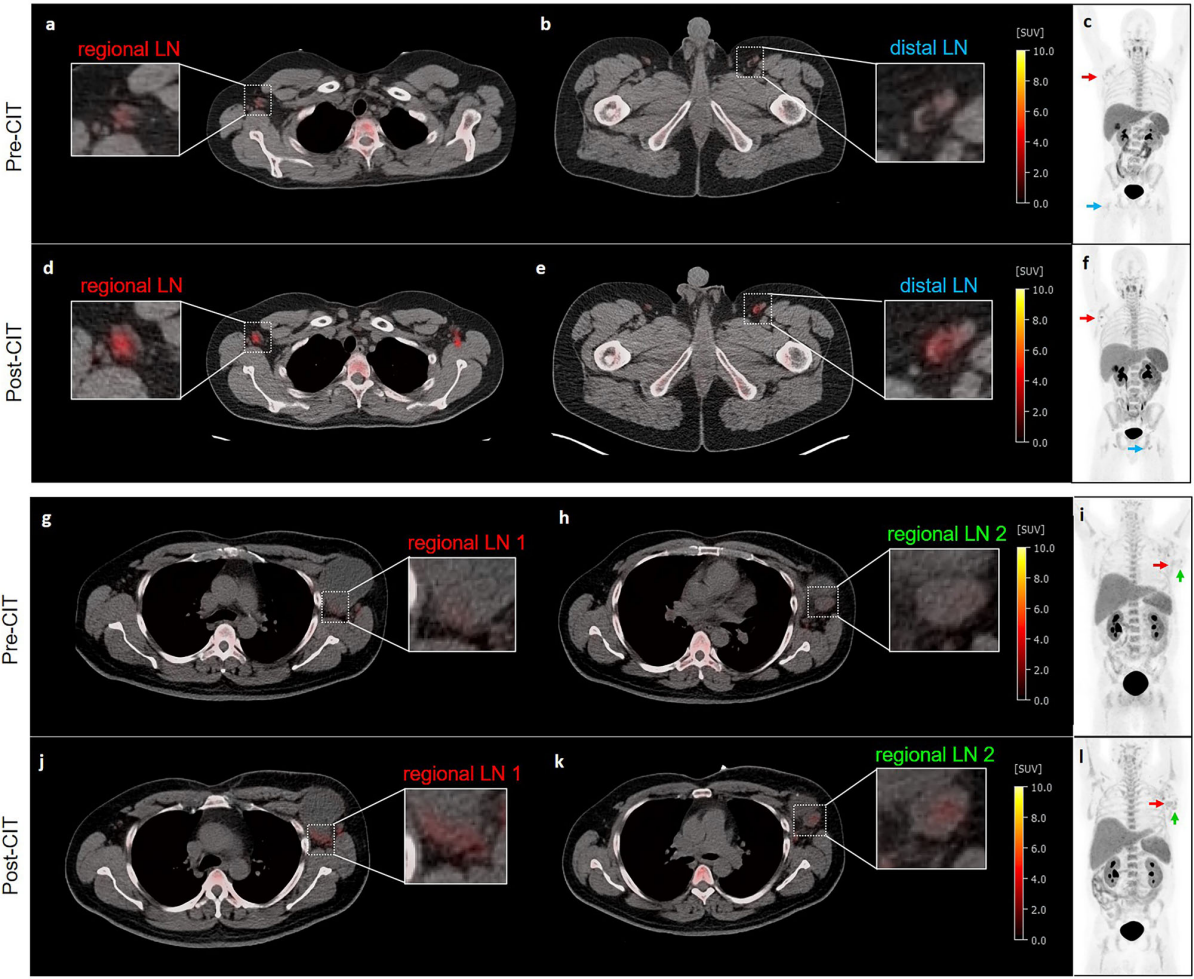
[^18^F]CFA-PET/CT in metastatic melanoma patients before and after CIT. 27-year-old male with subcentimeter lung lesions: baseline axial images showing (a) regional and (b) distal lymph node (LN) uptake of [^18^F]CFA. c Baseline maximum intensity projection image (MIP). d Axial, (e) distal LN uptake and f MIP after immune checkpoint inhibitor therapy (CIT) (red arrow: regional LN, blue arrow: distal LN). 47-year-old male patient with advanced melanoma and left axillary lymph node metastases: baseline axial images showing (g, h) regional LN uptake of [^18^F]CFA. i Baseline MIP. j, k Axial LN uptake and l MIP after immune checkpoint inhibitor therapy (CIT) (red arrow: regional LN 1, green arrow: regional LN 2).
